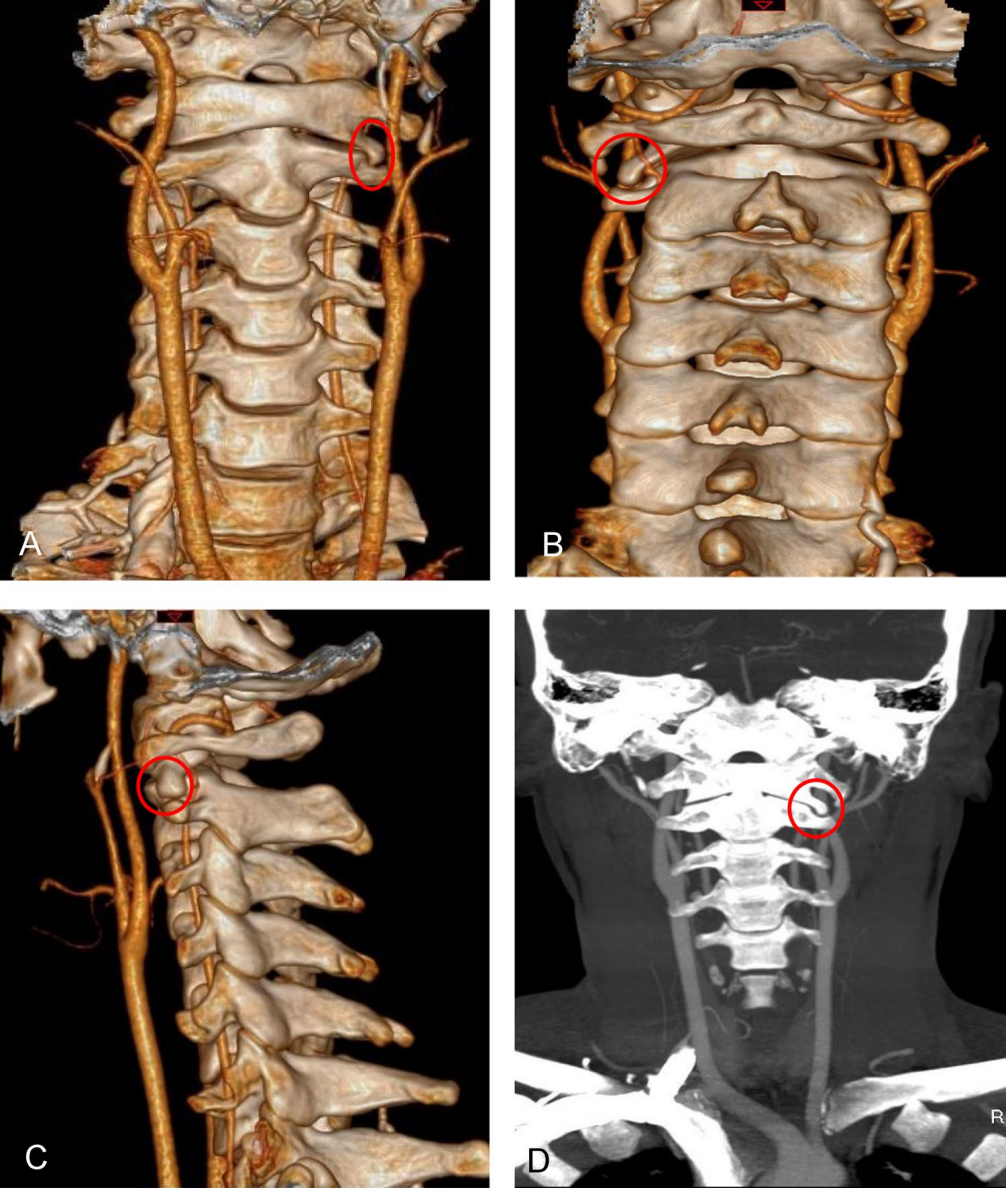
Preoperative 3D-computed tomography and Computed tomography angiography. Preoperative 3D-computed tomography (A, B, C) of the patient’s neck showing the precise anatomical location of the C1 left osteophyte (red circle). Preoperative Computed tomography angiography (D) of the patient’s neck showed no sign of bilateral vertebral artery stenosis in the neutral position, and the C1 left osteophyte (red circle) could be seen (Color figure online)
